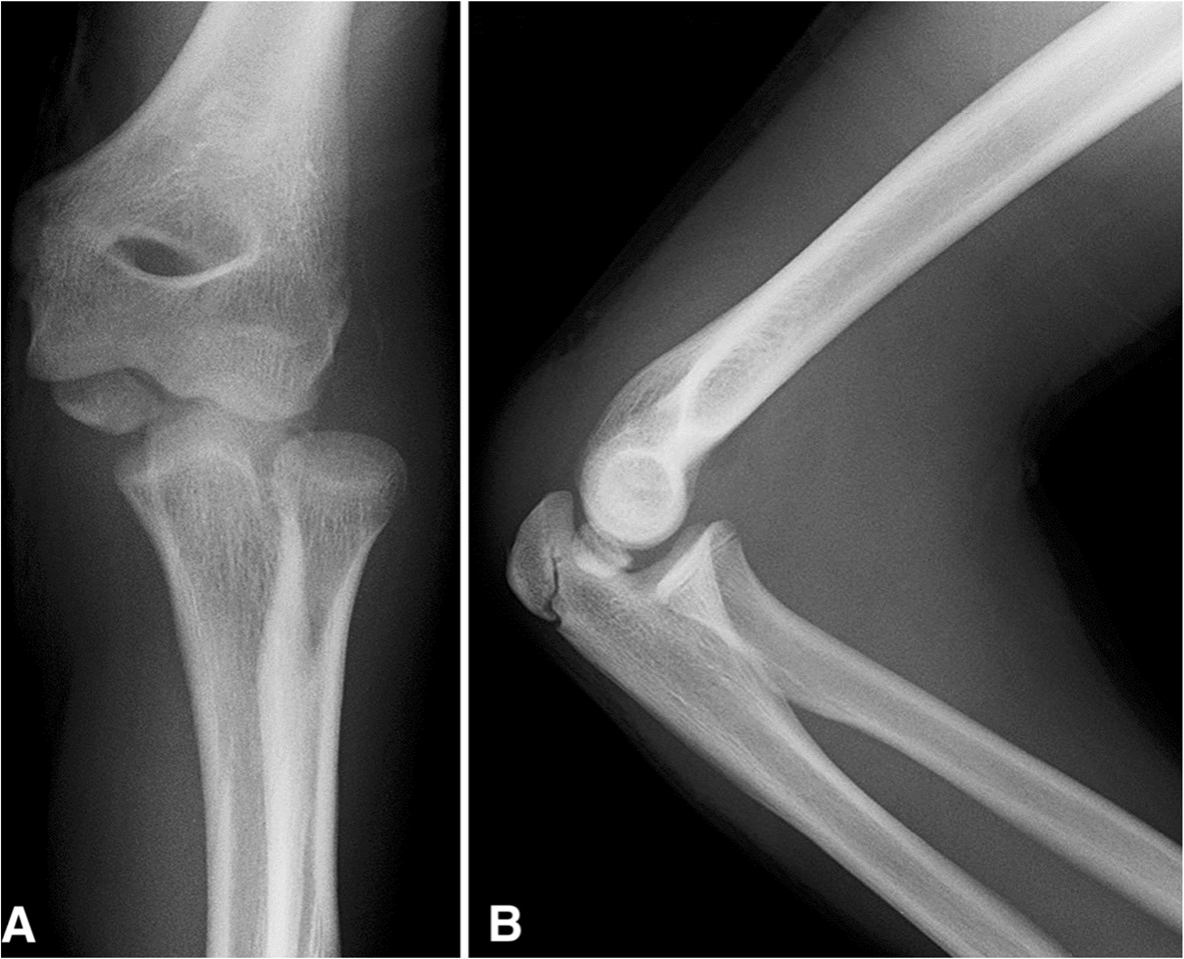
Anteroposterior radiograph (1A) and lateral radiograph (1B) of the right elbow demonstrating incarcerated medial epicondyle in the elbow joint following closed reduction.
